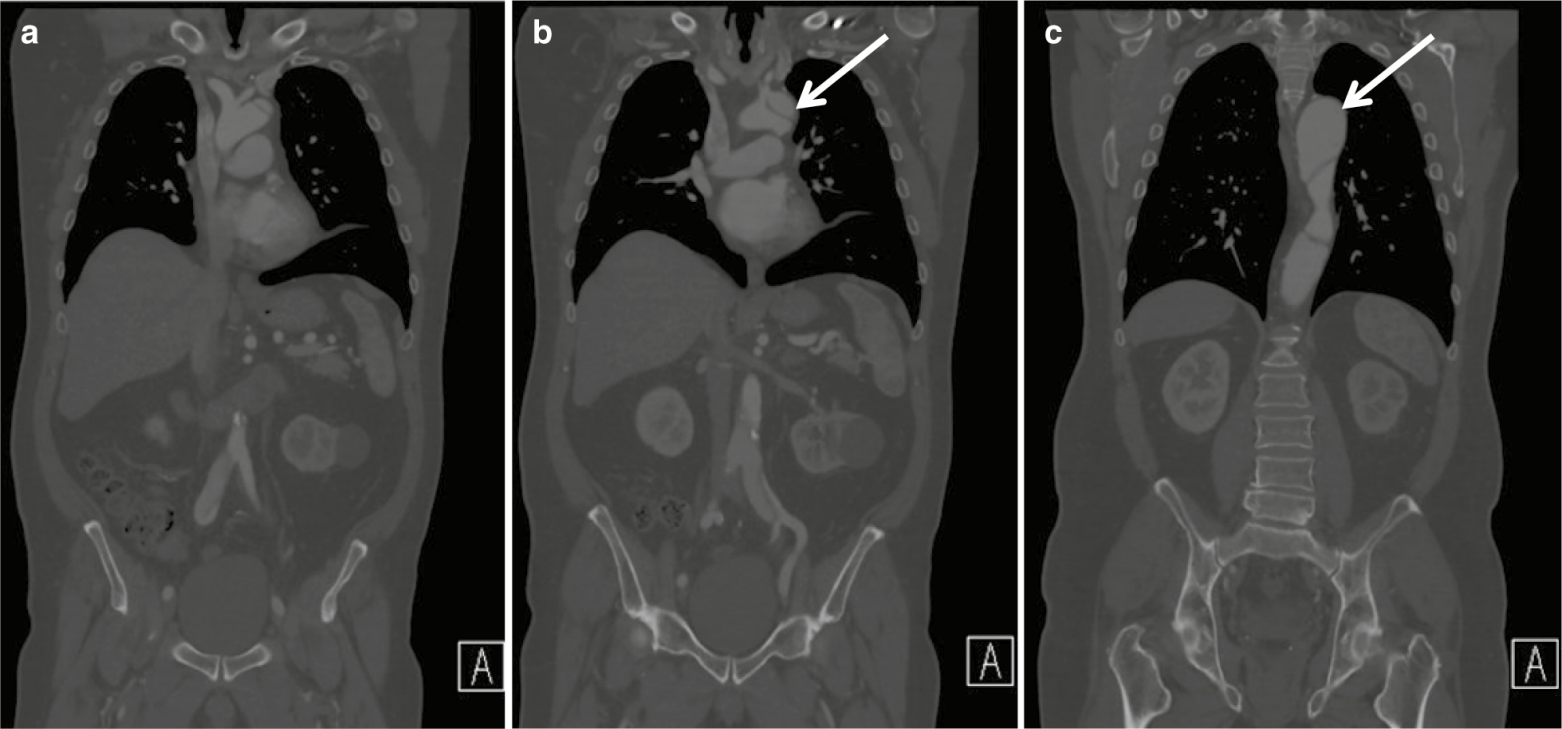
Surveillance computed tomography angiography coronal cuts from anterior to posterior (a–c, respectively). Arrows correspond to a chronic type B dissection involving the ostium of the left subclavian artery with aneurysmal degeneration to 5.3 cm. Distally, there is extension into the iliac bifurcation. There are multiple thoracic fenestrations, with all visceral vessels off the true lumen
